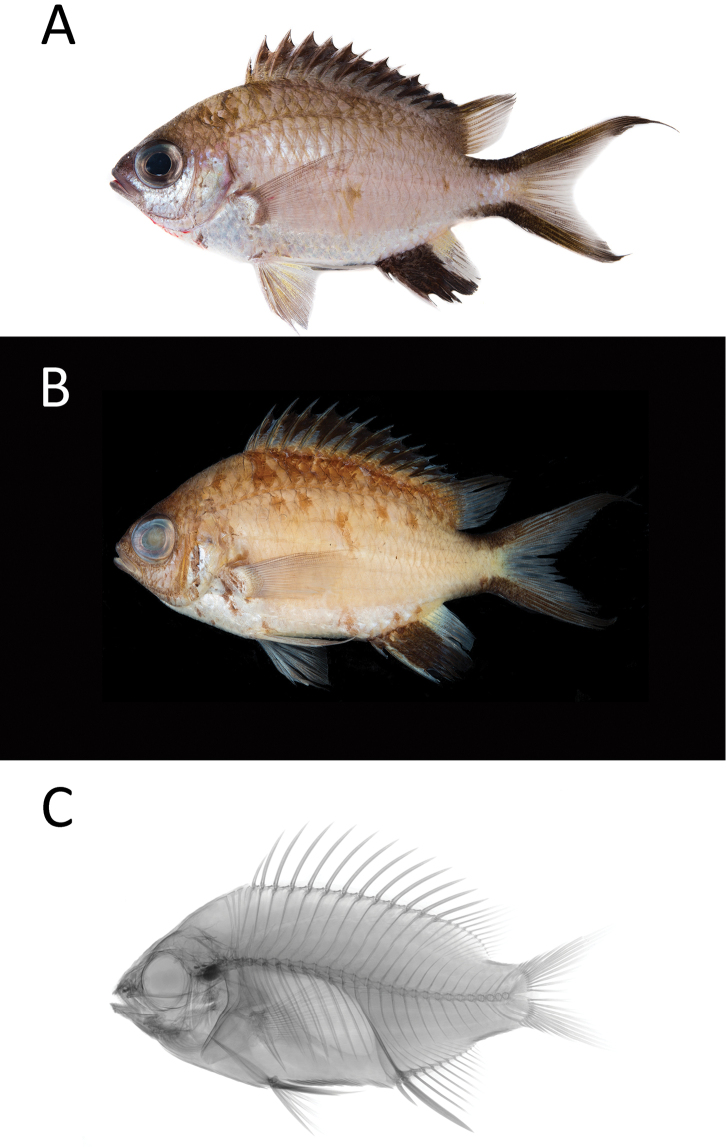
Chromisgunting* sp. n. PNM 15357 a holotype shortly after death, 67.69 mm SL, photograph LA Rocha b preserved holotype, photograph JD Fong c radiograph of holotype by JD Fong.
